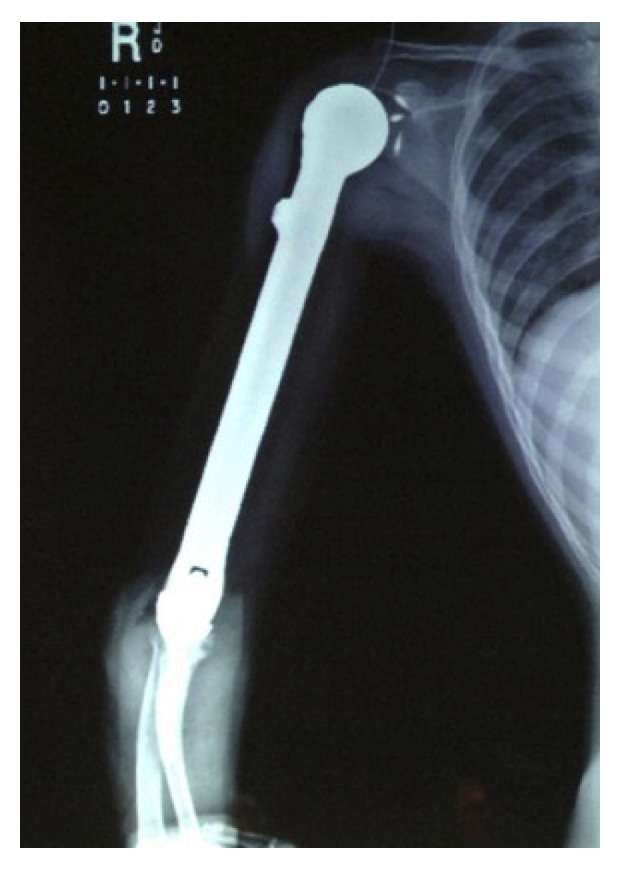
Postoperative anteroposterior humeral radiograph with implanted endoprosthesis.
